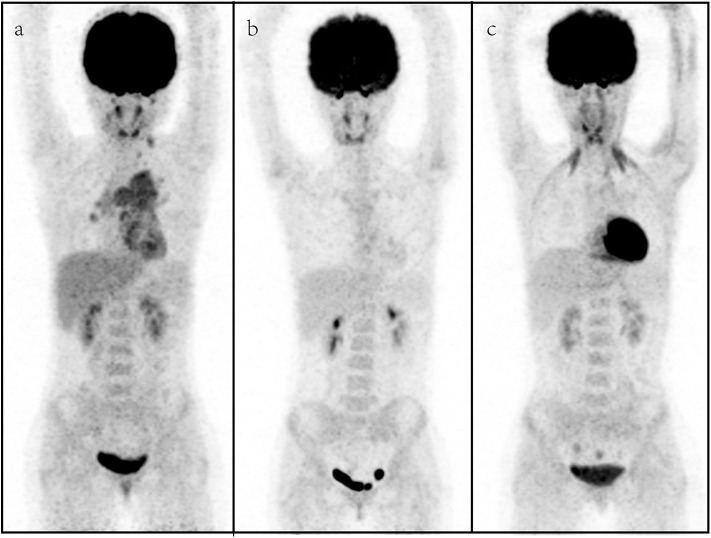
A representative case of an 11-year-old female with rhabdomyosarcoma. Baseline maximum intensity projection (MIP, a), showing hypermetabolic disease in laterocervical, mediastinal, and hilar lymph nodes, liver, and sternum [maximum standardized uptake volume (SUV_max_): 4.9, SUV_mean_: 3.4, SUV_peak_: 4.1, metabolic tumor volume (MTV): 77.2 cm^3^, total lesion glycolysis (TLG): 229.4]. PET/CT (b) after therapy showing a complete metabolic response with no fluoro-deoxyglucose (FDG) uptake in the previous sites. PET/CT (c) for follow-up revealed no FDG uptake lesions.
